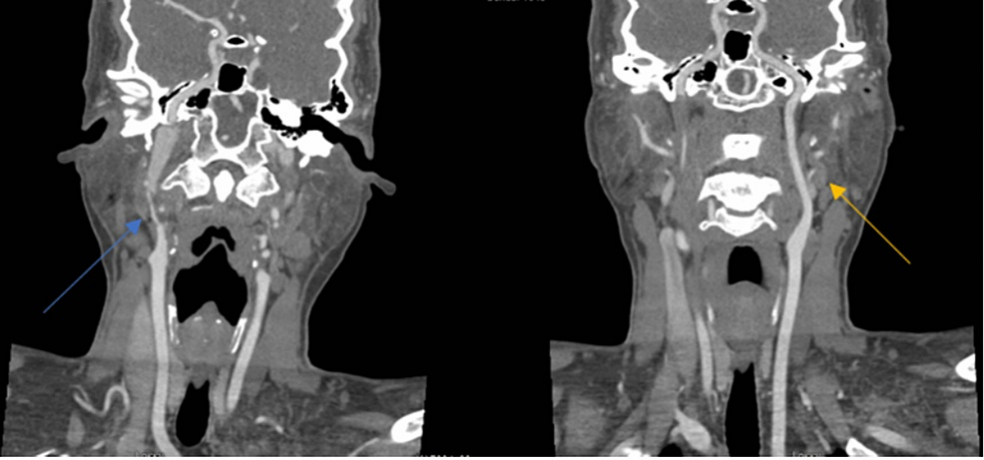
Computed tomography angiogram of the head and neck. The blue arrow represents the severe irregular stenosis of the cervical right internal carotid artery from shortly distal to its origin to its entering into the petrous portion with near-complete occlusion at the skull base. The yellow arrow shows the intact left carotid.
